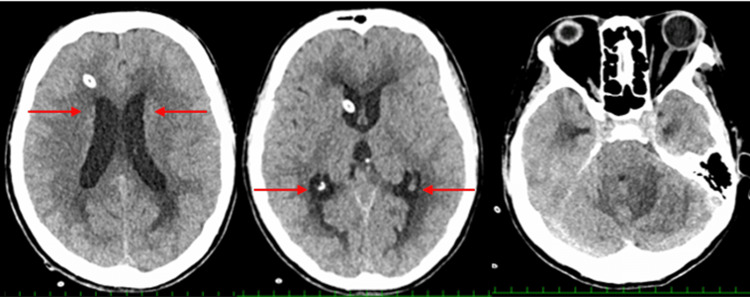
Non-Contrast Brain Computed Tomography (CT) on the Fifth Day of Hospitalization Follow-up brain CT showed a reduction in low-density areas, along with improvements in brain edema and hydrocephalus (red arrow).
